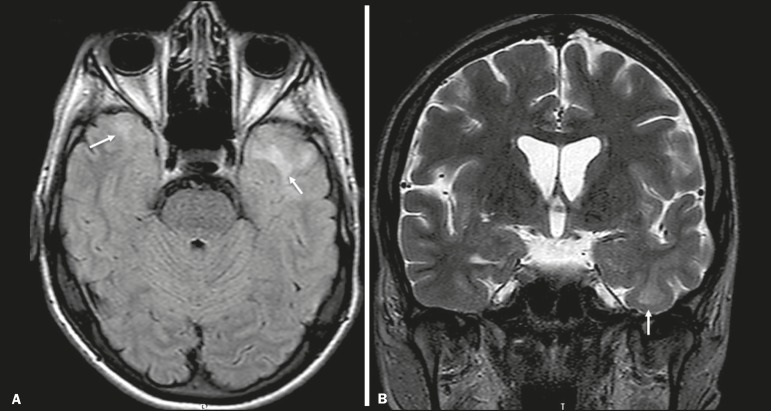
MRI of myotonic dystrophy type 1. A: Axial FLAIR sequence showing a hyperintense signal in the white matter of the temporal lobes. B: Coronal T2-weighted sequence showing an area of hyperintense signal in the white matter of the temporal lobes. There was no restricted diffusion on DWI (not shown).
